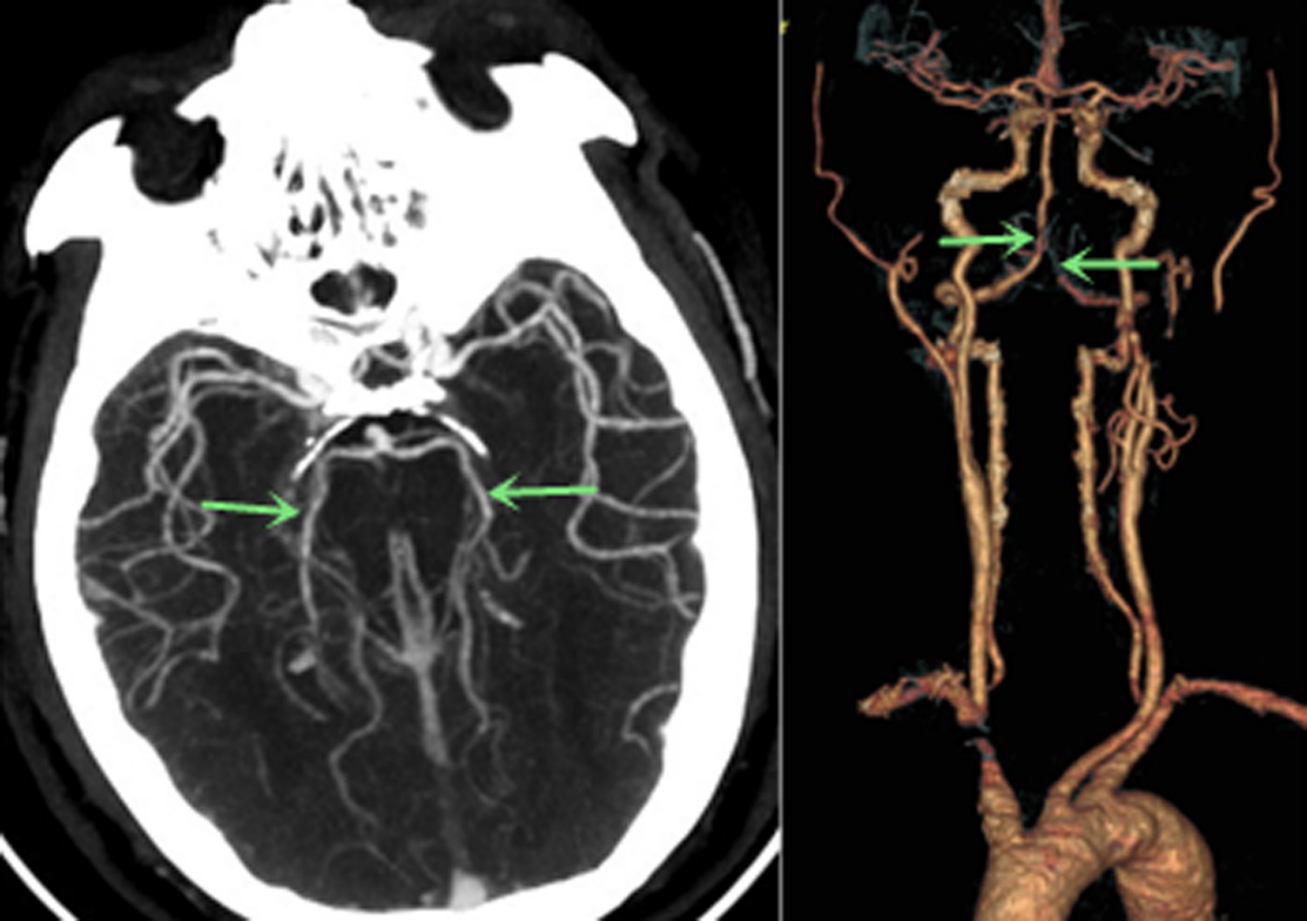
Admission CTA (225 min after symptom onset) showed significant stenosis in the basilar artery, and occlusion of the left V4 segment of the vertebral artery(arrow). Both bilateral posterior cerebral arteries were patent at this time (arrow).
